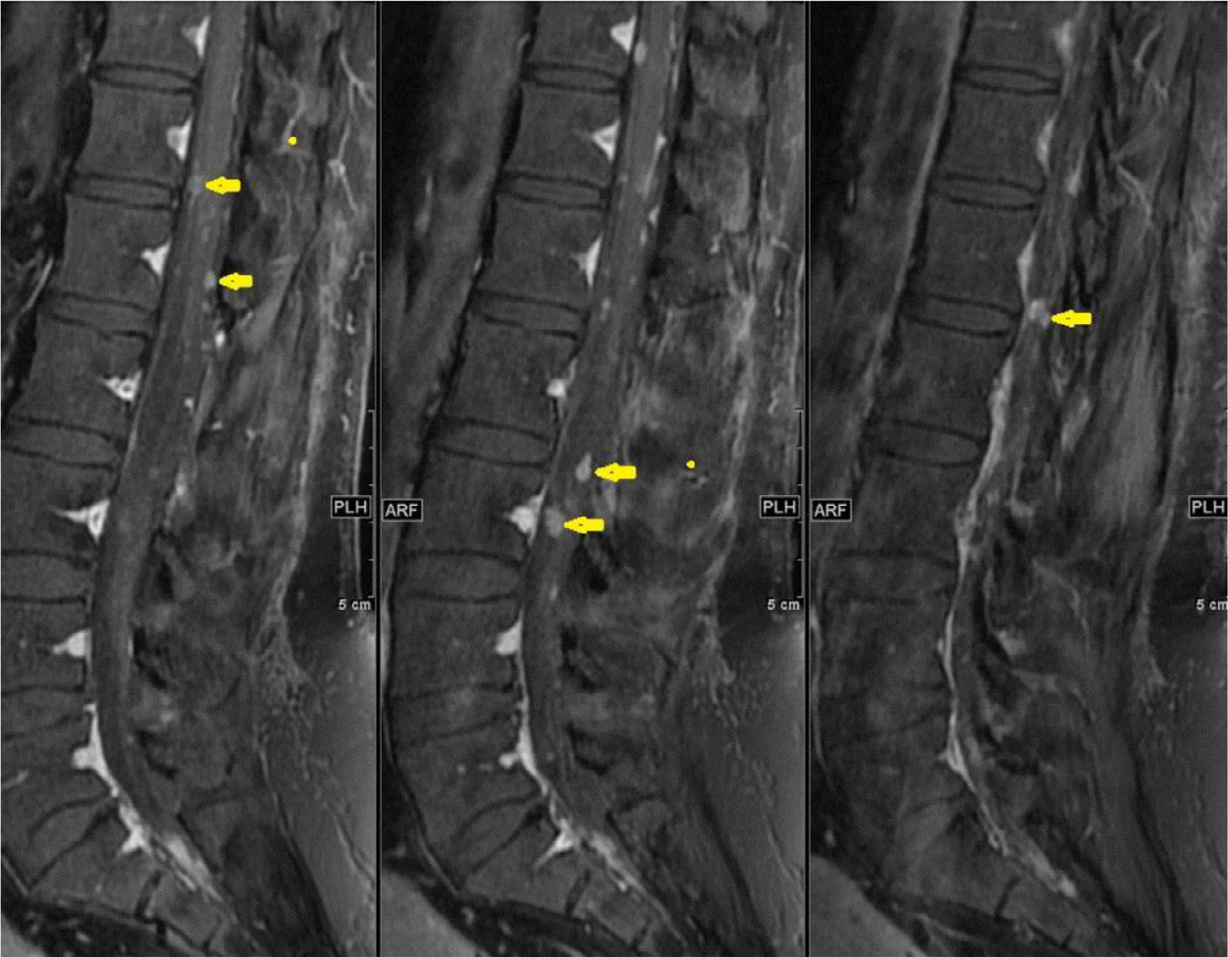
Sagittal MR images 48-months post-surgery of lumbar spine show increased number and size of the multiple enhancing intradural extramedullary nodules (arrows)
